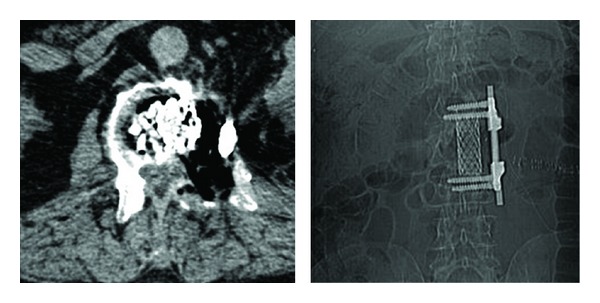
Postoperative CT scan demonstrating the tumor removal and the anterior column reconstruction.
